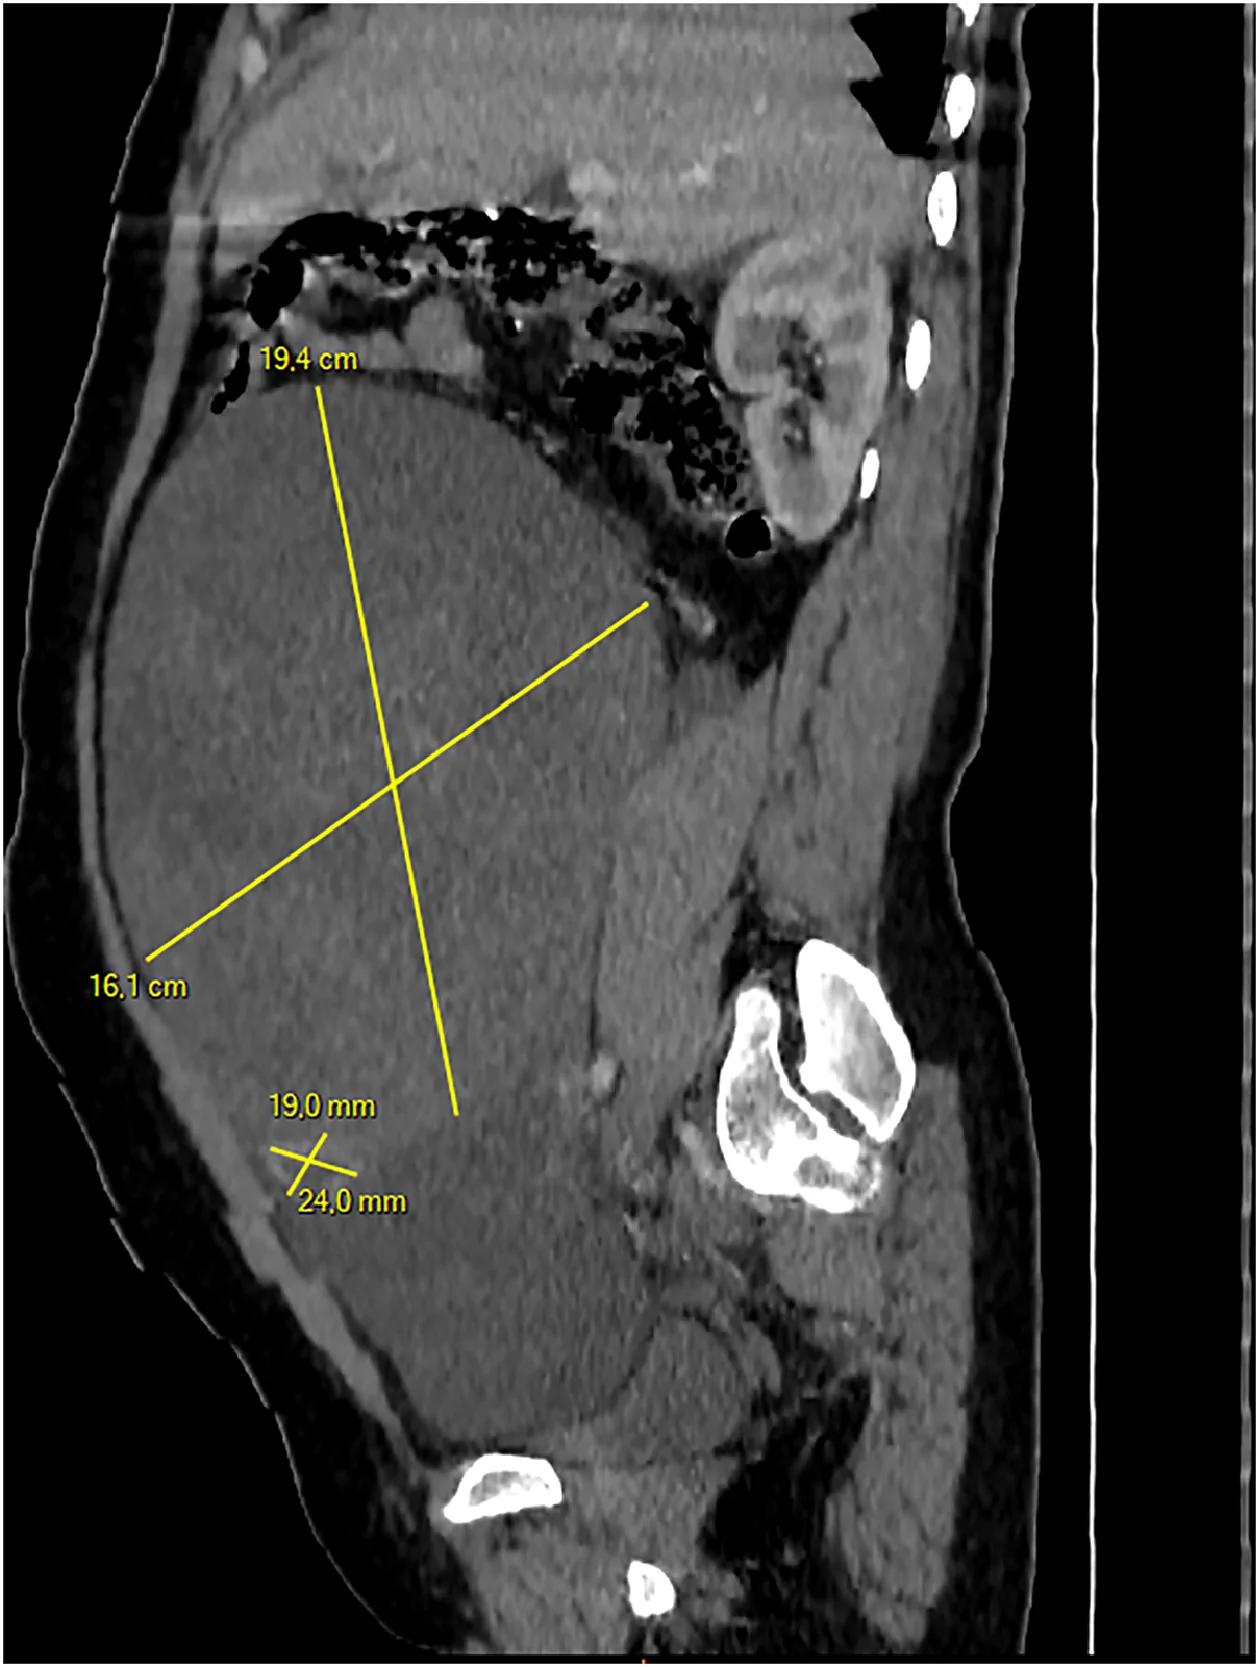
Frontal CT reconstruction demonstrating the giant ovarian mass associated with abundant ascites and inferior nodular peritoneal abnormality.
